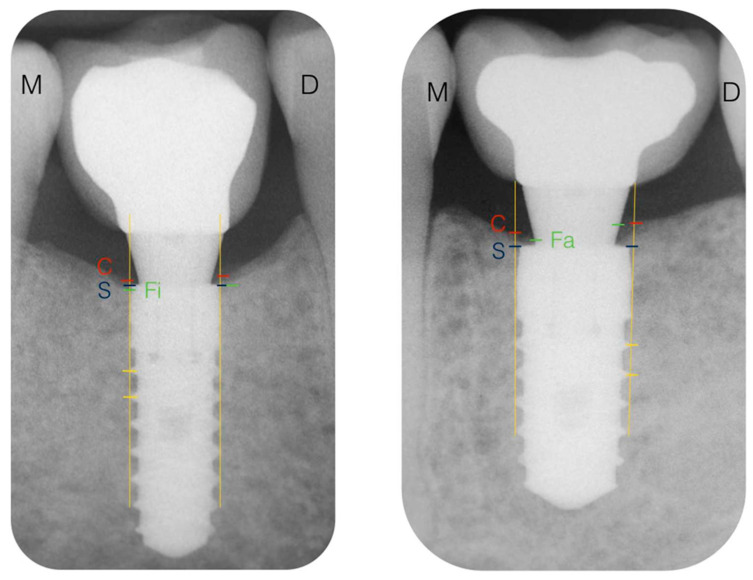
Reference landmarks in the X-rays. C, bone crest; S, implant shoulder; Fi, first bone–implant contact; M, mesial; D, distal; S–C distance, marginal bone level at the crest (MBLc); S–Fi distance, marginal bone level at the implant (MBLi); S–Fa distance, marginal bone level at the abutment (MBLa).
